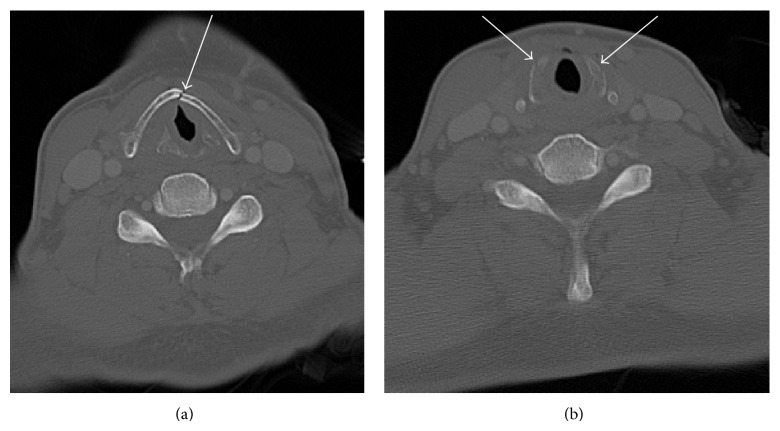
(a) Case A: axial contrast CT of the neck shows acute fracture of the mid anterior thyroid cartilage. (b) Case A: axial contrast CT of the neck shows acute comminuted fracture of both aspects of the cricoid cartilage.
